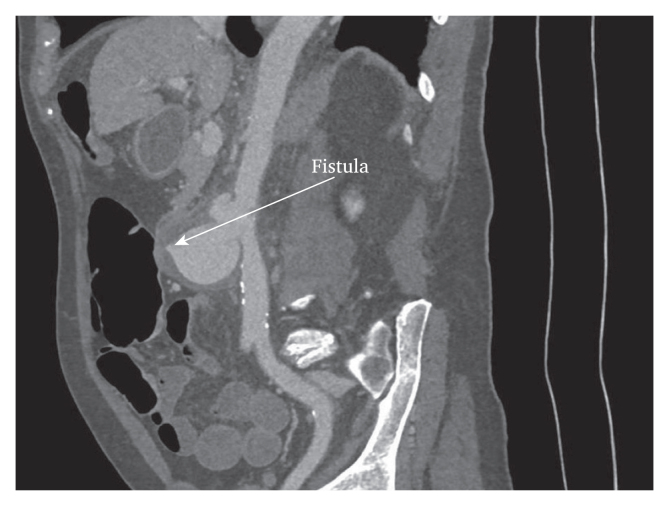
Multiple planar reconstruction of a computed tomography scan showing a large aortic aneurysm and primary aorto-enteric fistula (arrow).
